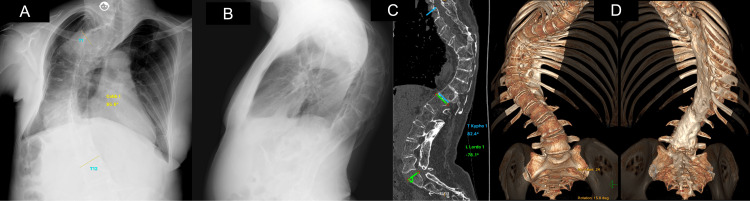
Imaging of the patient's spine (A) Anteroposterior Radiograph depicting scoliosis severity. (B) Lateral Radiograph depicting kyphosis severity (C, D) 3-Dimensional Computed Tomography of Spine. Parameters to indicate the severity of scoliosis have been annotated.
